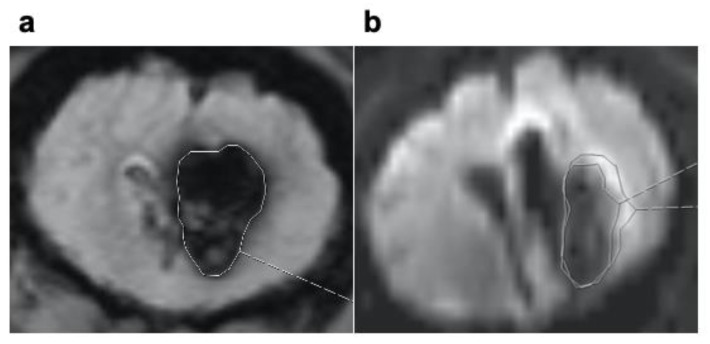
Cranial MRI a T2*- weighted image of (a) right frontal ICH white framed using the Sectra^®^ software (Linköping, Sweden). (b) DWI-image of a right frontal ICH. Diffusion restriction volume is white framed using the Sectra^®^ software.
